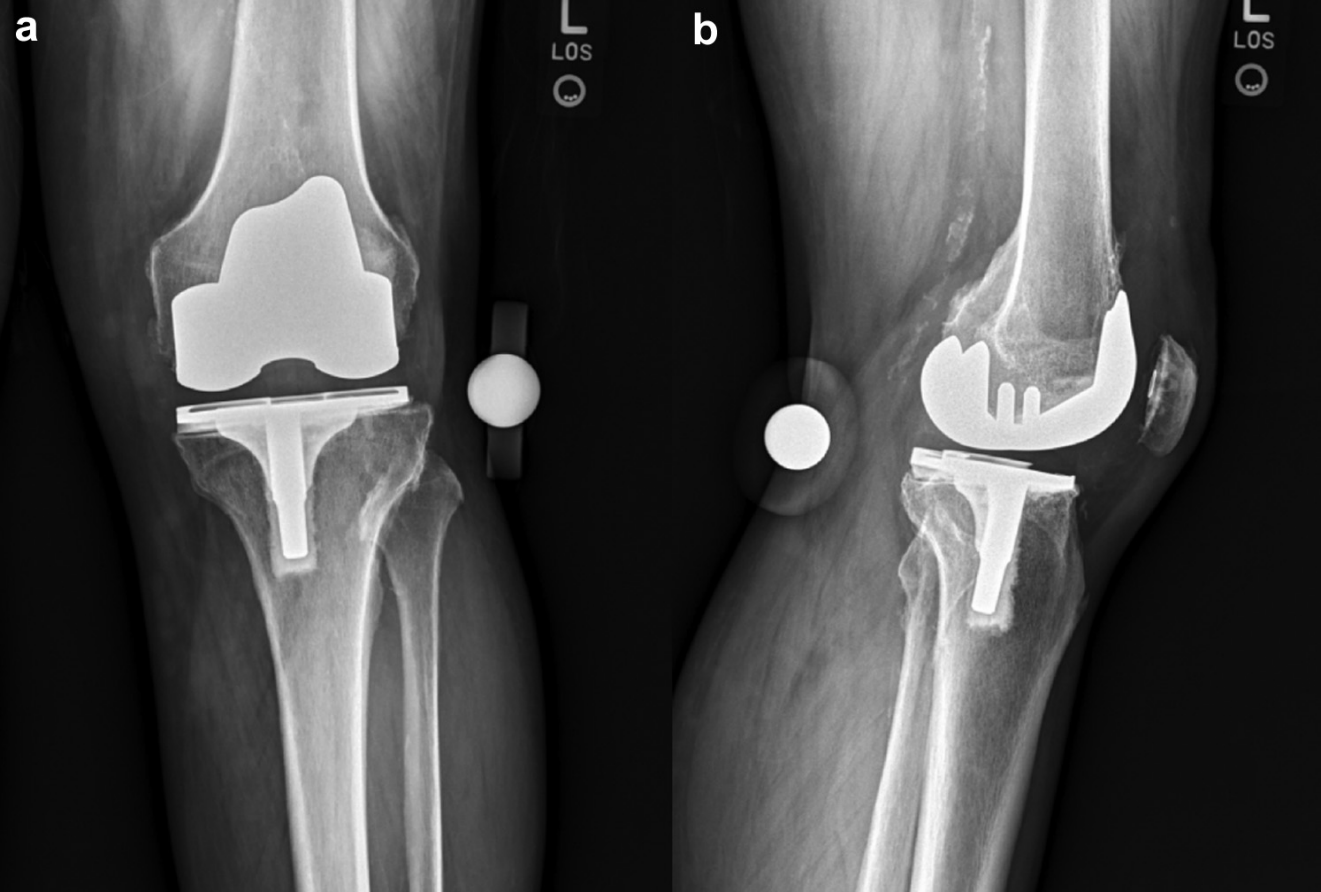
(a) Anteroposterior and (b) lateral radiographs of the left knee of case 2 demonstrate Smith and Nephew cruciate retaining design with components in reasonable alignment with lucency underneath the medial tibial baseplate. Vascular calcifications are noted. Decreased condylar offset seen on the lateral view without evidence of fracture.
